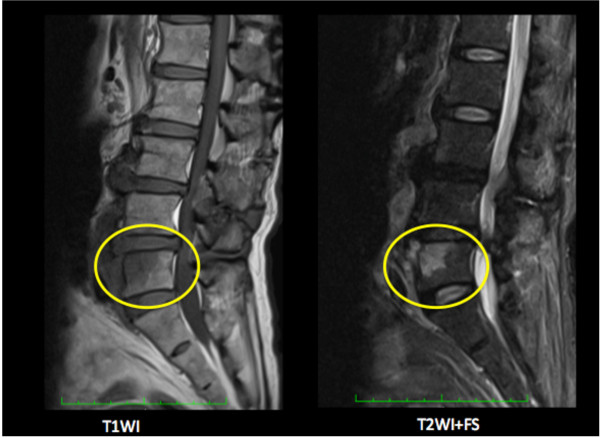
MRI in Case 1.*Circle*; Low-intensity area with unclear boundary on the T1-weighted image (*left*) and high intensity on the T2-weighted image + fat saturation (*right*) on the ventral side of the 5^th^ lumbar vertebral body.
